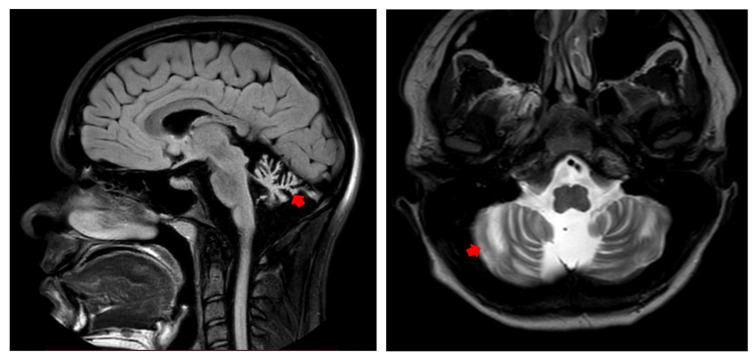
Brain MRI showing severe atrophic changes in the white matter of the cerebellum, primarily in the vermis (red arrows).
